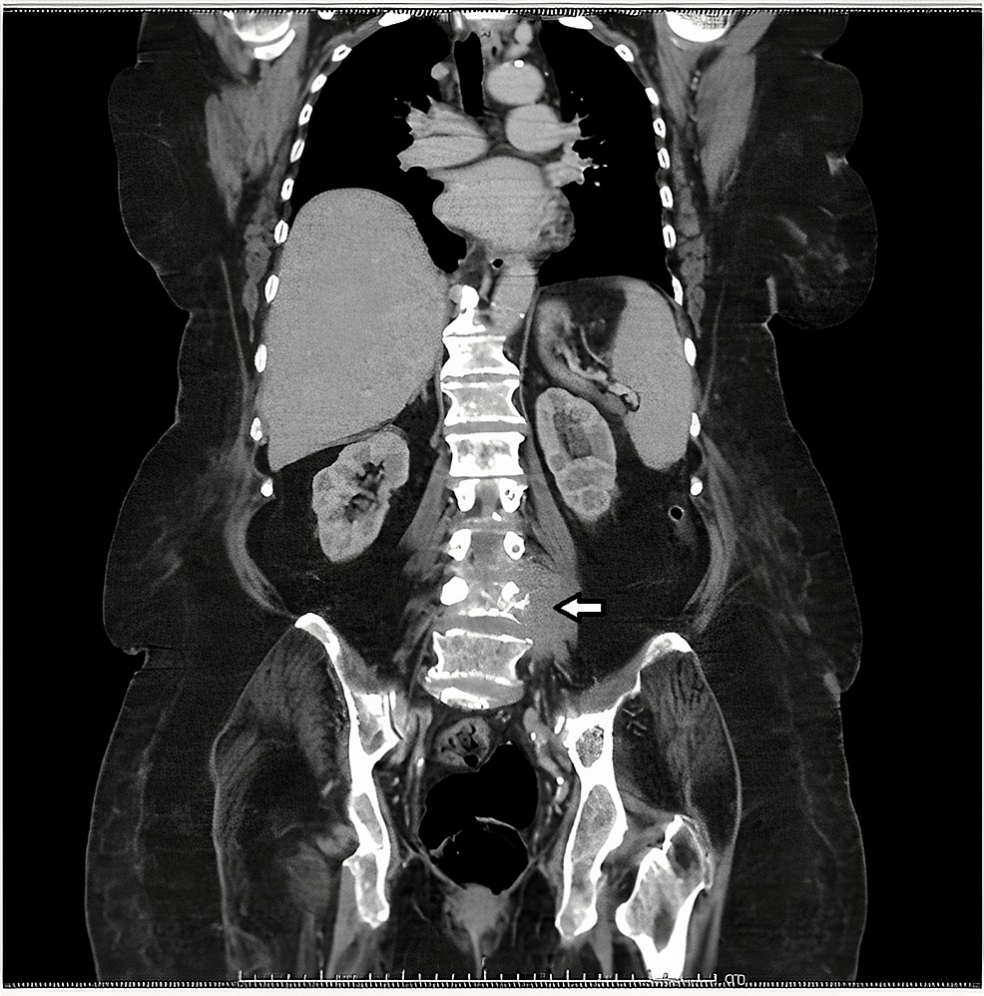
Thoraco-abdominopelvic computed tomography scan. Large mass component centered on the vertebral body of L4 and with greater left medial and anterior perivertebral expression (arrow), with signs of invasion of the psoas muscle and contacting the homolateral iliac vessels.
